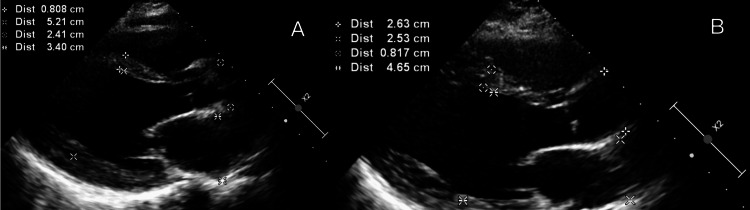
Parasternal long-axis view. (A) At diagnosis measuring left ventricular dimensions. (B) Improved left ventricular dimensions at the one-month follow-up.
